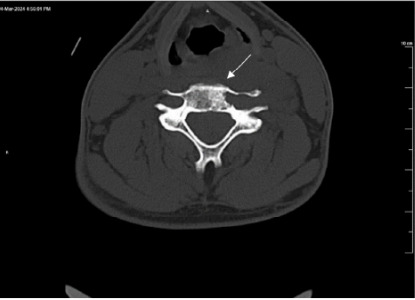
Transverse computed tomography of the cervical spine without contrast demonstrating lucent expansile lesion involving the fifth cervical vertebral body and transverse process (arrow), consistent with Ewing sarcoma.
